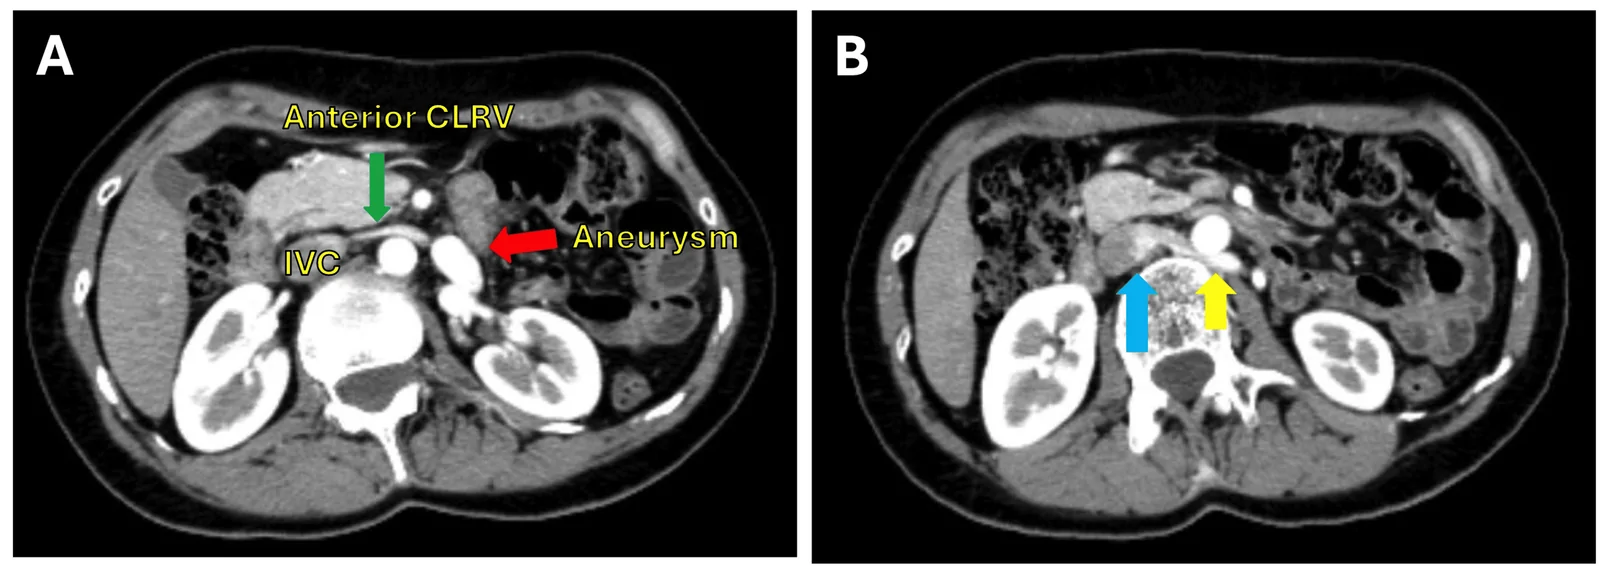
Contrast-enhanced computed tomography (CECT) of the abdomen. (A) Axial view demonstrating a circumaortic left renal vein (CLRV). The anterior limb (green arrow) courses anterior to the abdominal aorta to drain into the inferior vena cava (IVC), with an associated saccular venous aneurysm (red arrow). (B) Inferior axial slice confirming the posterior retroaortic limb (yellow arrow) passing posterior to the abdominal aorta toward its IVC confluence (blue arrow), without evidence of extrinsic compression or entrapment.
